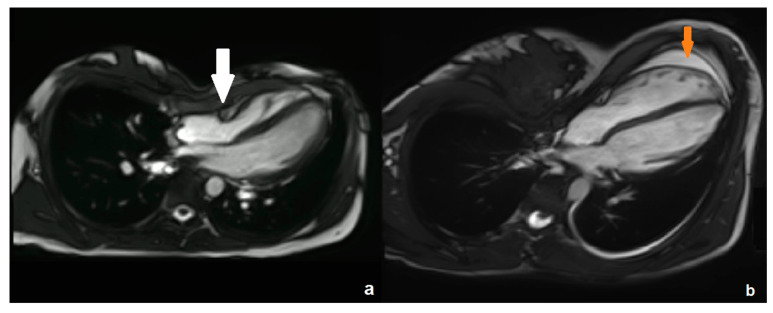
An example of a steady-state free-precession cardiac MRI sequence demonstrates the most frequent type of compression of the RV base by the sternum ((a), white arrow). In a second example (b), a shift of the entire heart to the left is depicted in addition to a pericardial effusion (orange arrow).
